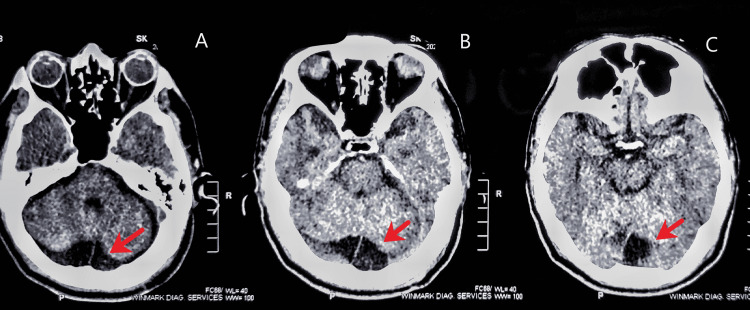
Axial sections of head CT showing retrocerebellar hypodense lesion
